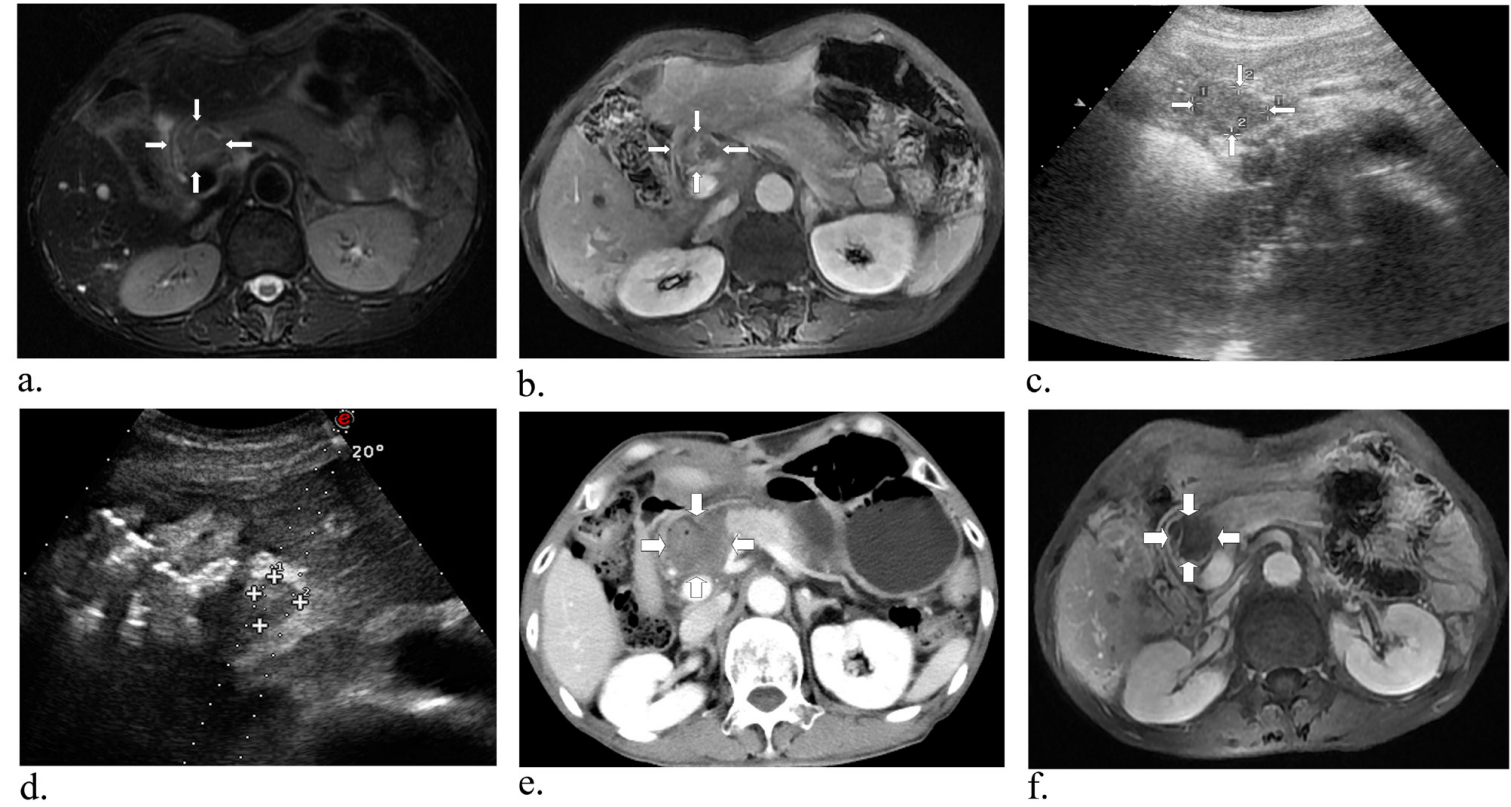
A 60-year-old man with metastatic lymph nodes close to the duodenum, pancreas, stomach and blood vessels Preoperative T2-weighted a. (white arrows), substance phase MR scan indicated a tumor close to the hepatic portal vein, pancreas and stomach b. (white arrows). Axial US image of the retroperitoneal region showed the mild hyperechoic area c. (white arrows). After fourth ablation, US image showed the lesion had complete response d. (white arrows). Substance phase of CT obtained 3 days after US-guided PLA revealed no signs of malignancy e. (white arrows), and then one month later, substance phase of MR image has low signal intensity f. showing complete necrosis of the tumour (white arrows).
